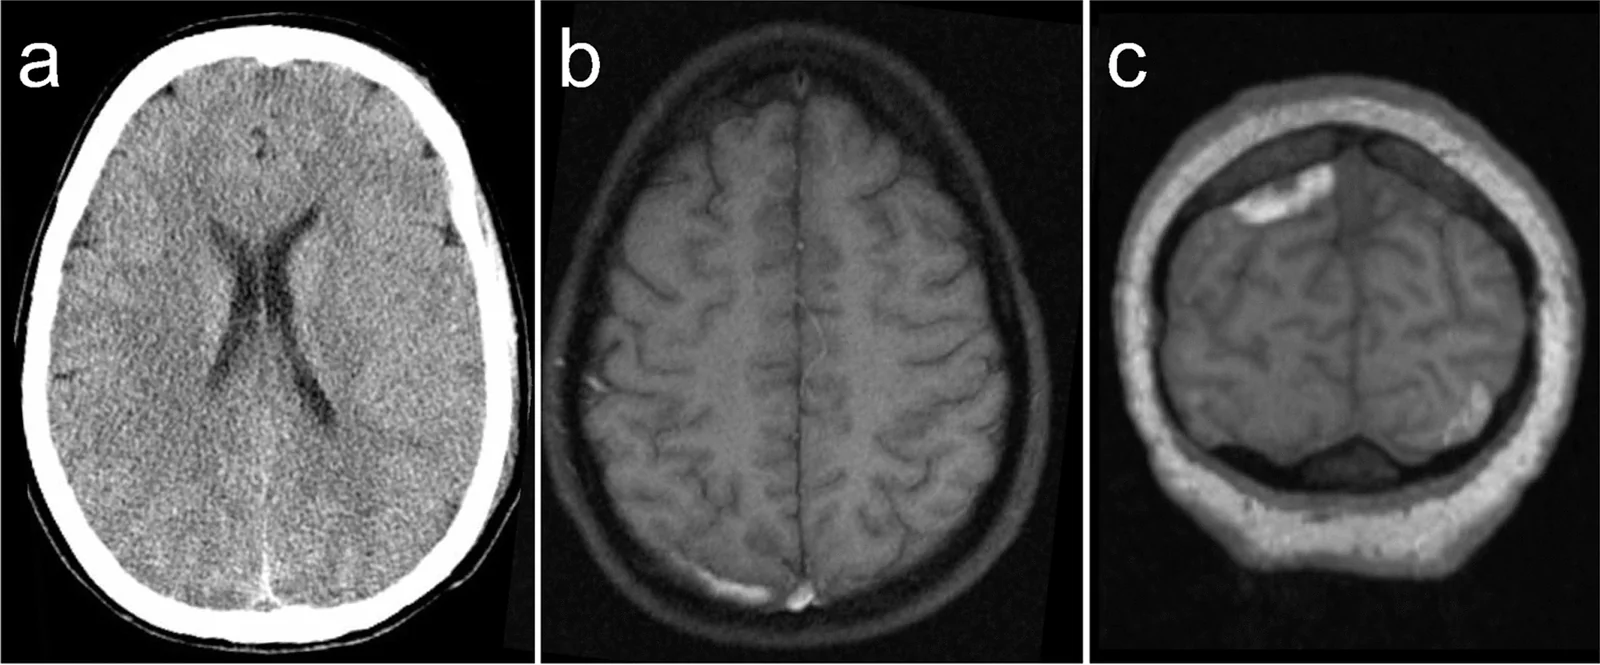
Neuroimaging case #2. (a) CT scan performed three hours after the injury revealing slight occipital brain edema. Axial (b) and coronal section at level of the occipital and parietal lobe (c) T1-weighted images conducted six days after LI demonstrated occipital subarachnoid bleeding[14]
